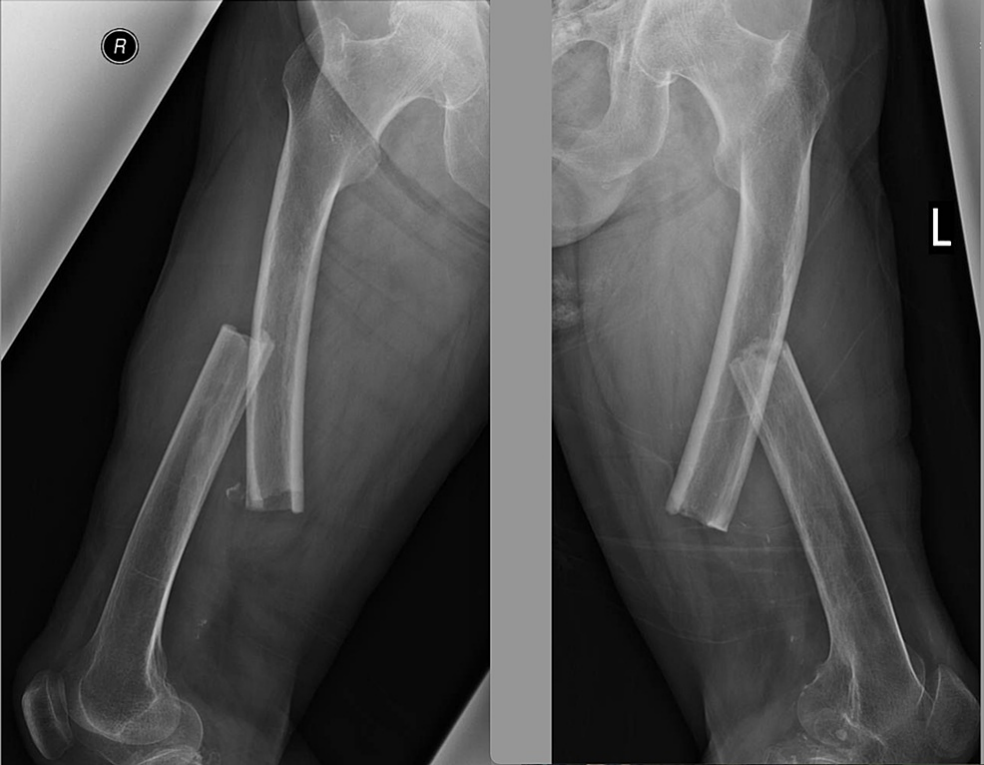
Plain radiographs of the right and left femurs. Bilateral transverse fractures with cortical thickening suggest the fractures were atypical.
